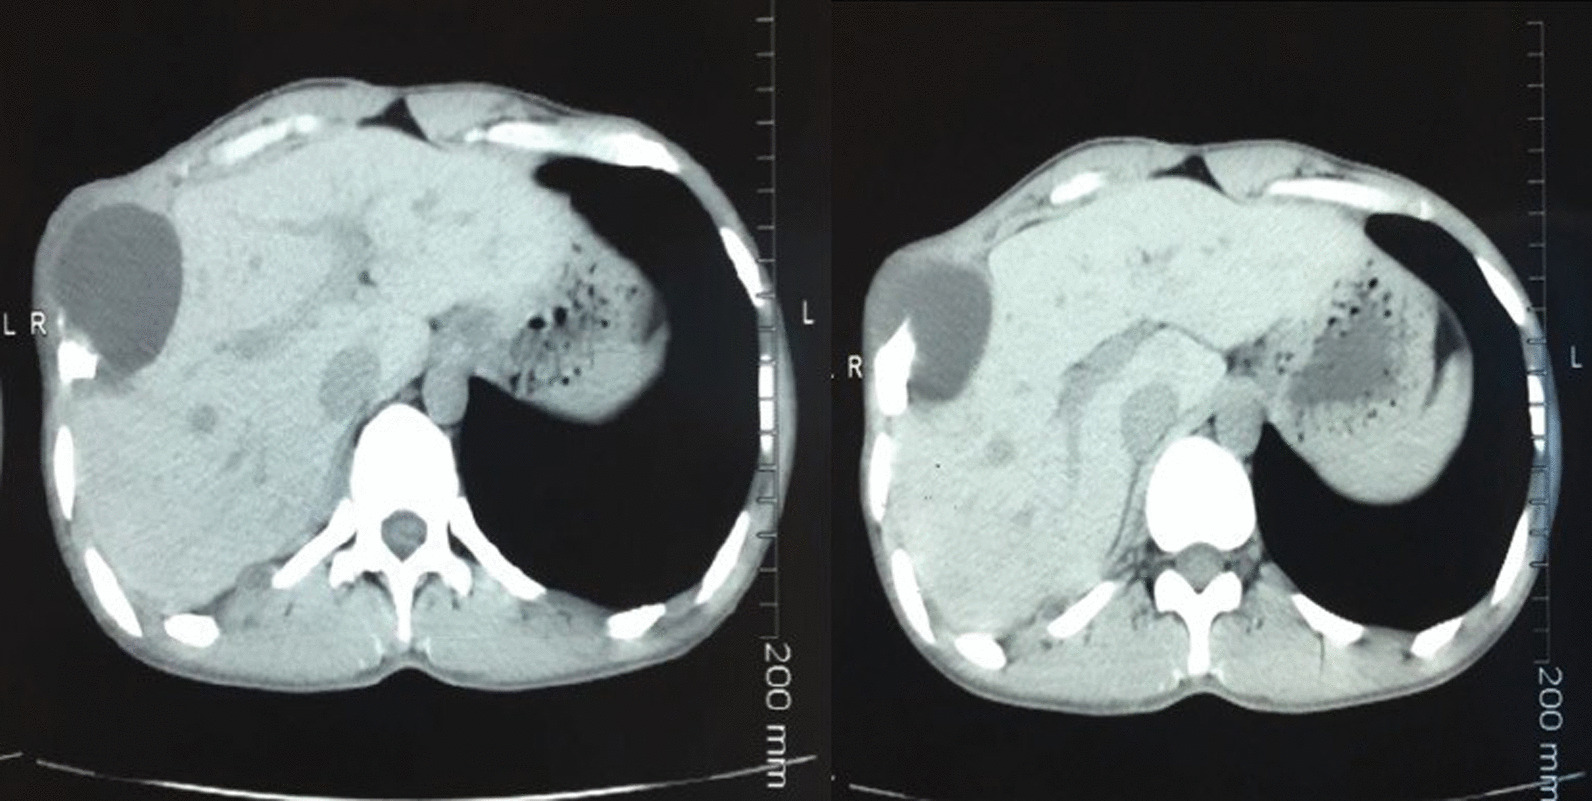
Thoraco-abdominal CT scan showing an a latero-basal thoracic hydatid cyst, measuring 5 cm in large diameter, invading the soft parts and the lateral arch of the 8th rib, without hepatic impairment
